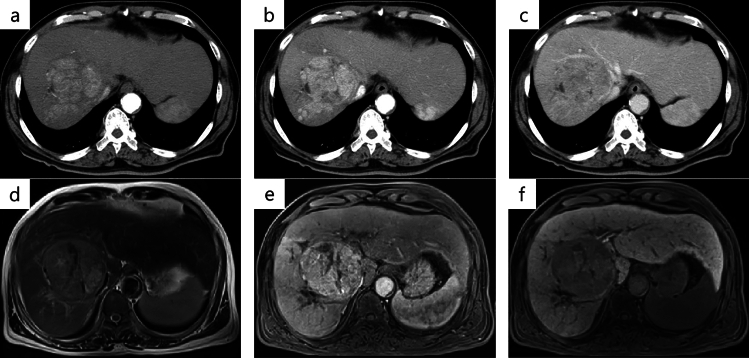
CT and MRI at first visit. a Arterial phase of dynamic CT. b Portal phase of dynamic CT. c Venous phase of dynamic CT. d T2-weighted image of MRI. e Arterial phase of EOB-MRI. f Hepatocyte phase of EOB-MRI. On dynamic CT, the tumor is early contrasted in the arterial phase and washed out in the venous phase. MRI shows a mildly high signal on T2-weighted images. In EOB-MRI, an early contrast effect and a low signal in the hepatocellular phase compared to surrounding normal hepatocytes
